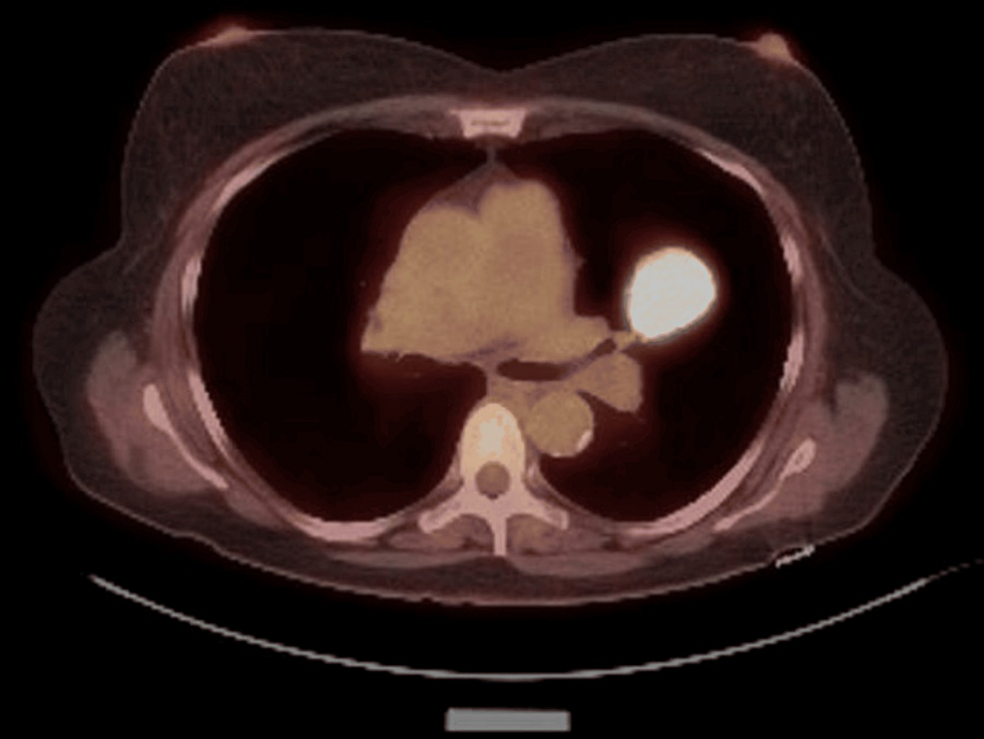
PET-CT scan: a moderately hypermetabolic LUL mass (SUV = 16.2) consistent with bronchogenic carcinoma, with no mediastinal or hilar lymphadenopathy PET-CT: positron emission tomography-computed tomography, SUV: standard uptake value, LUL: left upper lobe.
